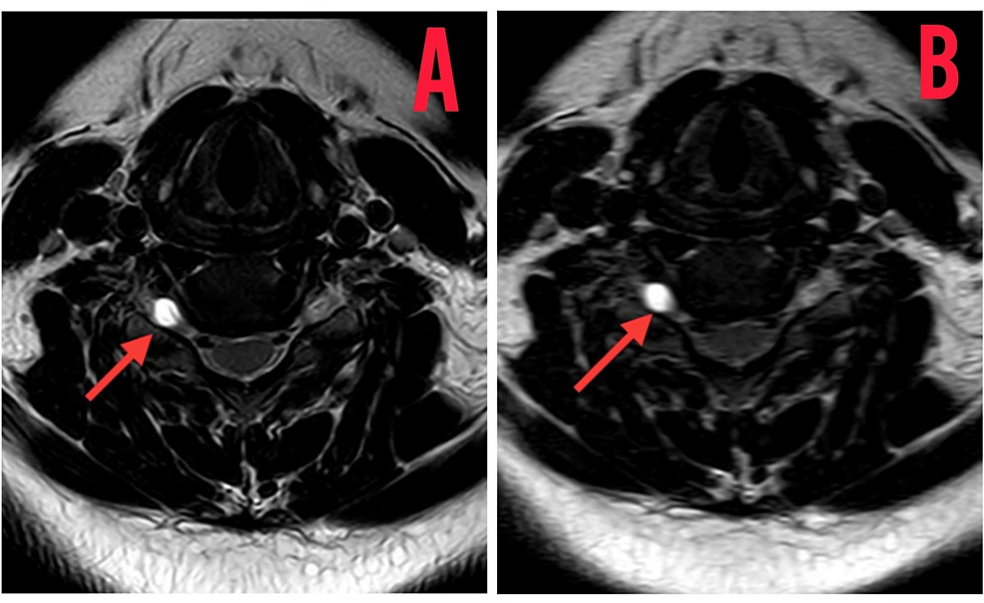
MRI of the cervical spine transverse view depicting a Tarlov cyst 5.91 mm in size MRI: Magnetic resonance imaging (A0 Tarlov cyst shadow in the transverse view; (B) hyperintense lesion of the Tarlov cyst
